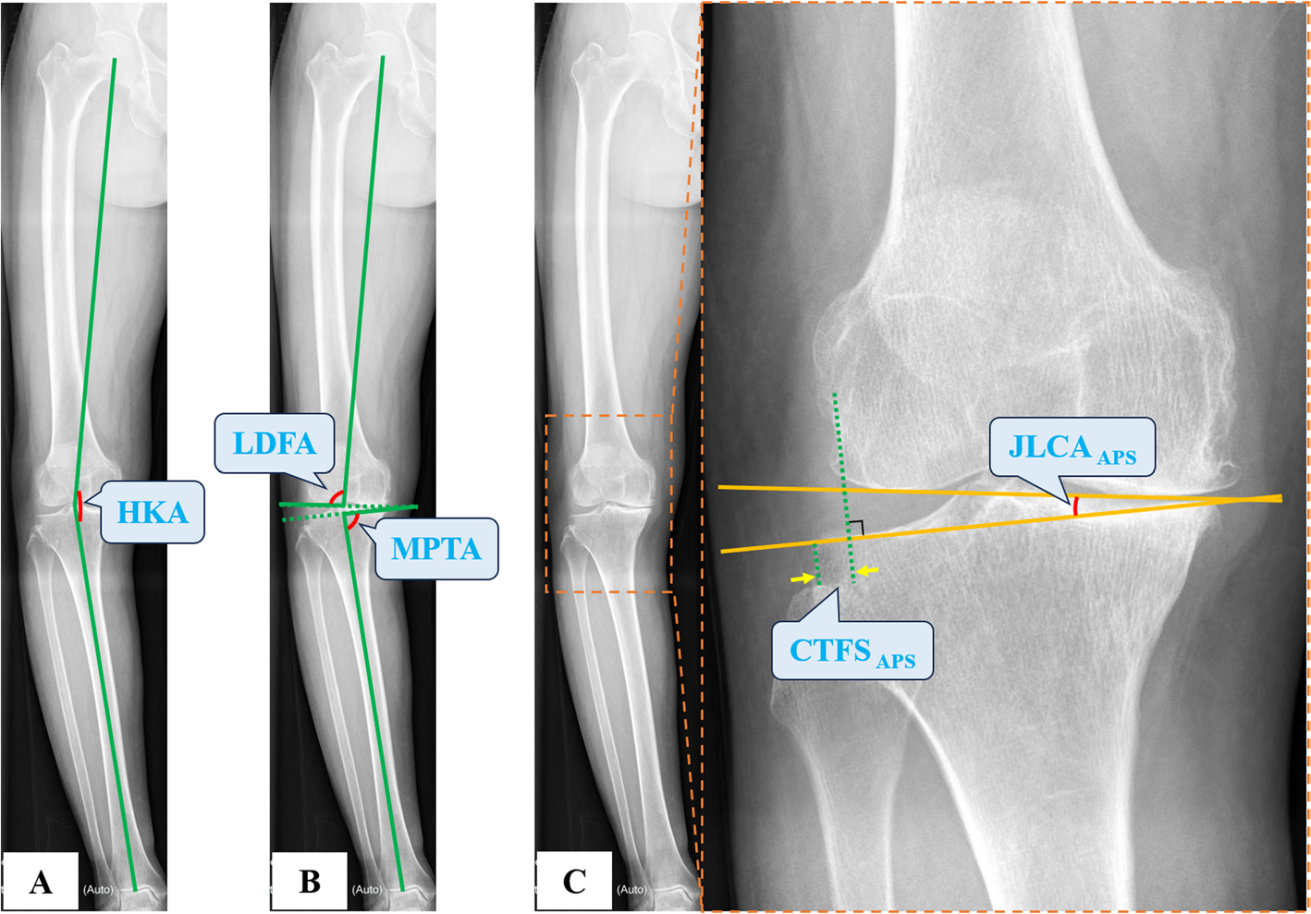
Measurement of parameters on the hip-to-ankle APS radiograph. A The midpoints of the femoral head, knee, and ankle joint were connected to form HKA. B LDFA was defined as the lateral angle subtended by the distal femoral condylar line and the femoral mechanical axis. MPTA referred to the medial angle between the tibial plateau line and the mechanical axis. C On the APS radiograph, CTFS_APS_ was recorded as the distance between the tangent line to the outermost joint edge of the lateral femoral condyle and the utmost margin of the lateral tibial plateau, and JLCA_APS_ referred to the angle between the distal femoral condylar line and the tibial plateau line
